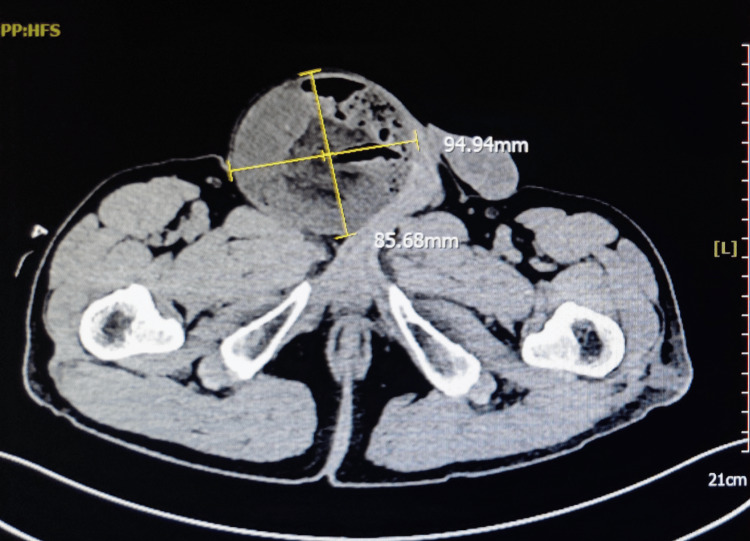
CT of the abdomen and pelvis CT of the abdomen and pelvis without contrast revealed a right-sided inguinal hernia with multiple loops of small bowel within the sac, with the largest cross-sectional area being approximately 9.4×8.8 cm
